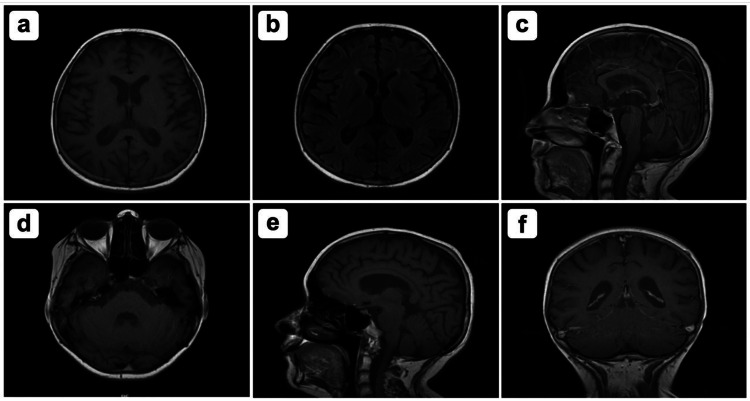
MRI with and without contrast in seven sections indicating: A) interval improvement of the T2 hyperintensity and resolution of the associated enhancement involving bilateral mammillary bodies and dorsal medulla. B) Interval resolution of the abnormal T2-flair hyperintensity involving periaqueductal region. C) Stable mild bilateral periventricular T2 hyperintensity. D) No new focal parenchymal signal abnormality. E) Stable mild diffuse parenchymal volume loss with enlargement of the extra-axial CSF spaces and ventricular system. No evolving hydrocephalus. F) Stable susceptibility artifact of the bilateral Globus Pallidi corresponding to the mineralization on prior brain CT. CSF: Cerebrospinal fluid
